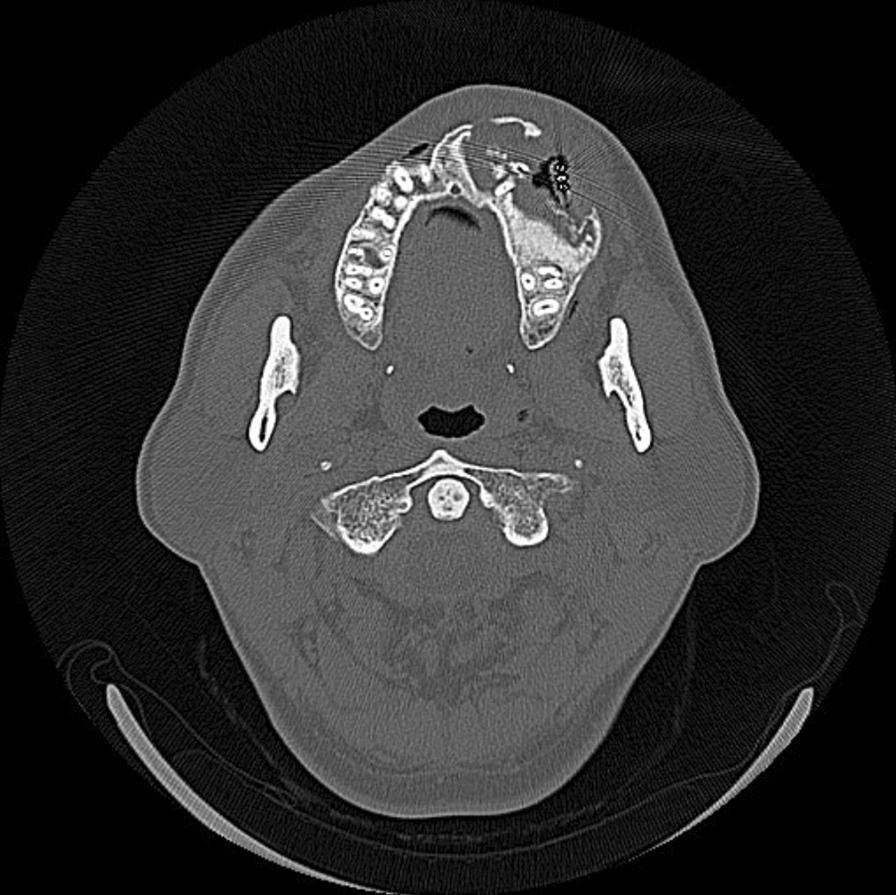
Computed tomography findings 1.5 years after marsupialization. Growth of radiopaque bone-like tissue was observed in the interior of the tumor, without metastases to the surrounding tissues
